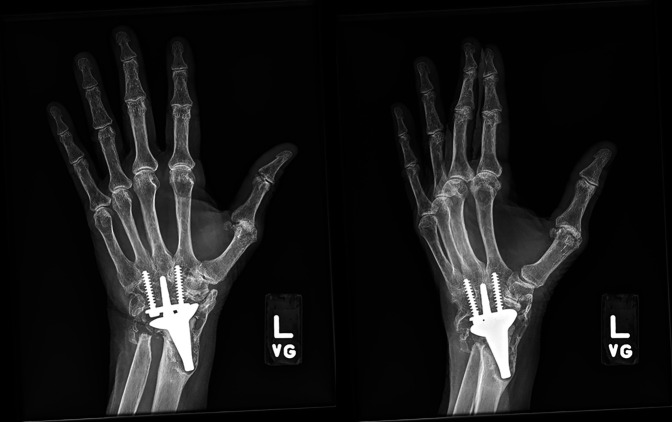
AP and oblique radiographs of the left wrist of a patient with total wrist arthroplasty demonstrating failure and loosening of the proximal component.
